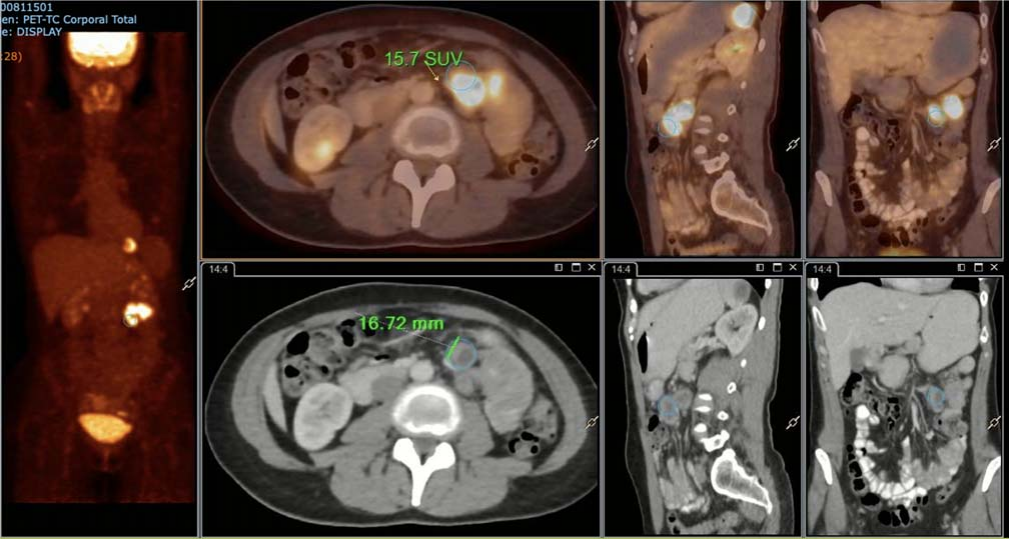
PET/CT showing a hypermetabolic peritumoral lymph node (SUVmax 15.7).
